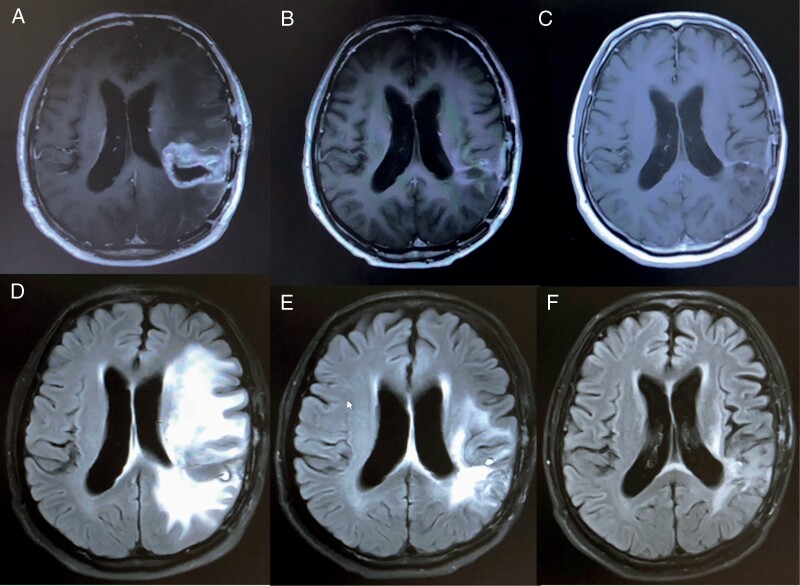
Brain MRI demonstrated recurrent *POLE*-mutated glioblastoma with partial response to pembrolizumab and bevacizumab. T1W + Gadolinium sequence in the upper panel (A-C) and T2W/FLAIR sequence in the lower panel (D-F). (A) and (D) at baseline; (B) and (E) at 3 months after pembrolizumab plus bevacizumab; and (C) and (F) at 12 months after pembrolizumab plus bevacizumab. T1W, T1-weighted; T2W, T2-weighted; FLAIR, Fluid-attenuated inversion recovery.
